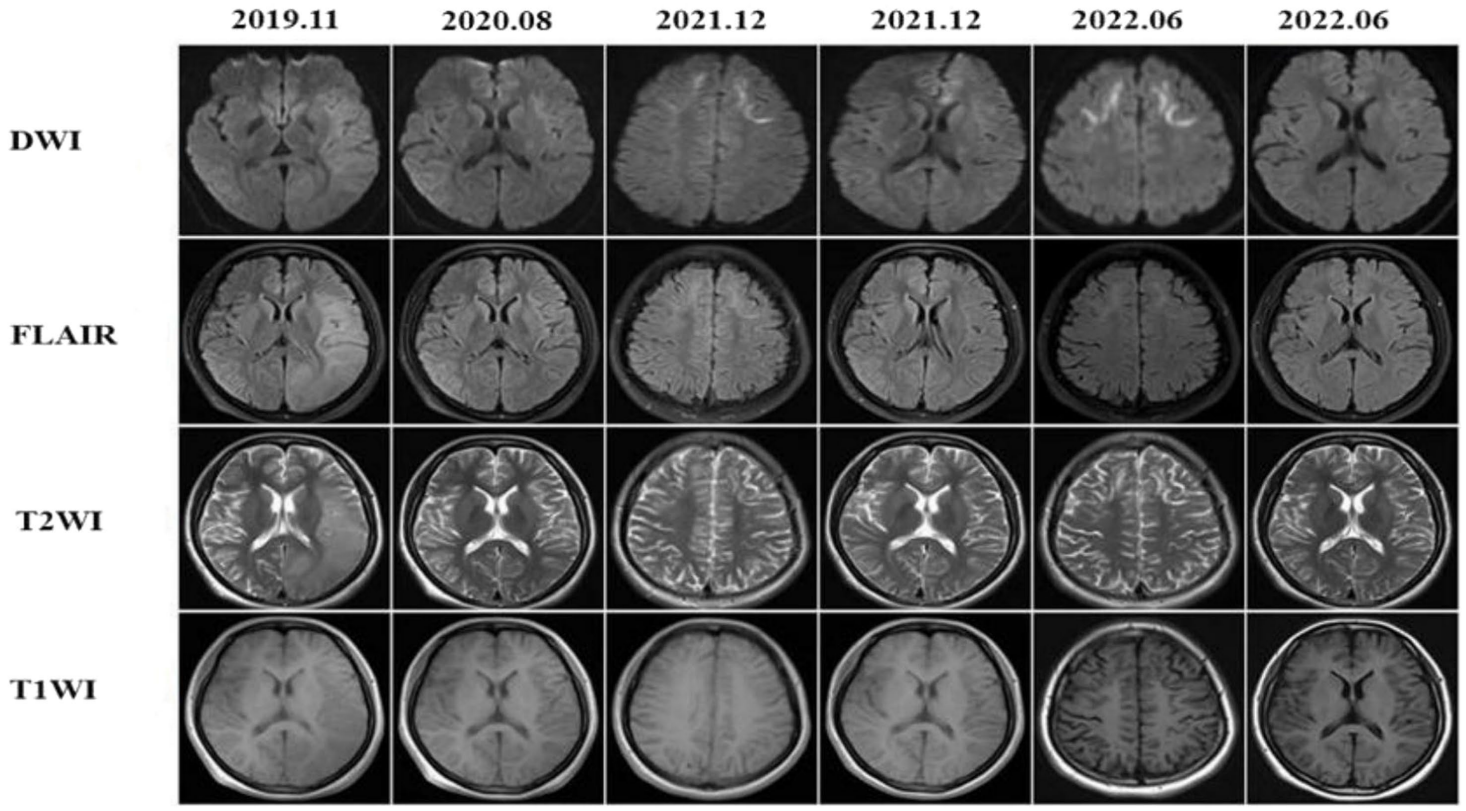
The patient presented with recurrent episodes of encephalitis with reversible cortical swelling on the left cerebral hemisphere in 2019 and the edema was completely recovered in 2020, but the typical high-intensities on DWI were observed at the bilateral frontal lobes, right parietal lobe and the corpus callosum in 2021. The lesions at the bilateral frontal lobes were more prominent in 2022.
